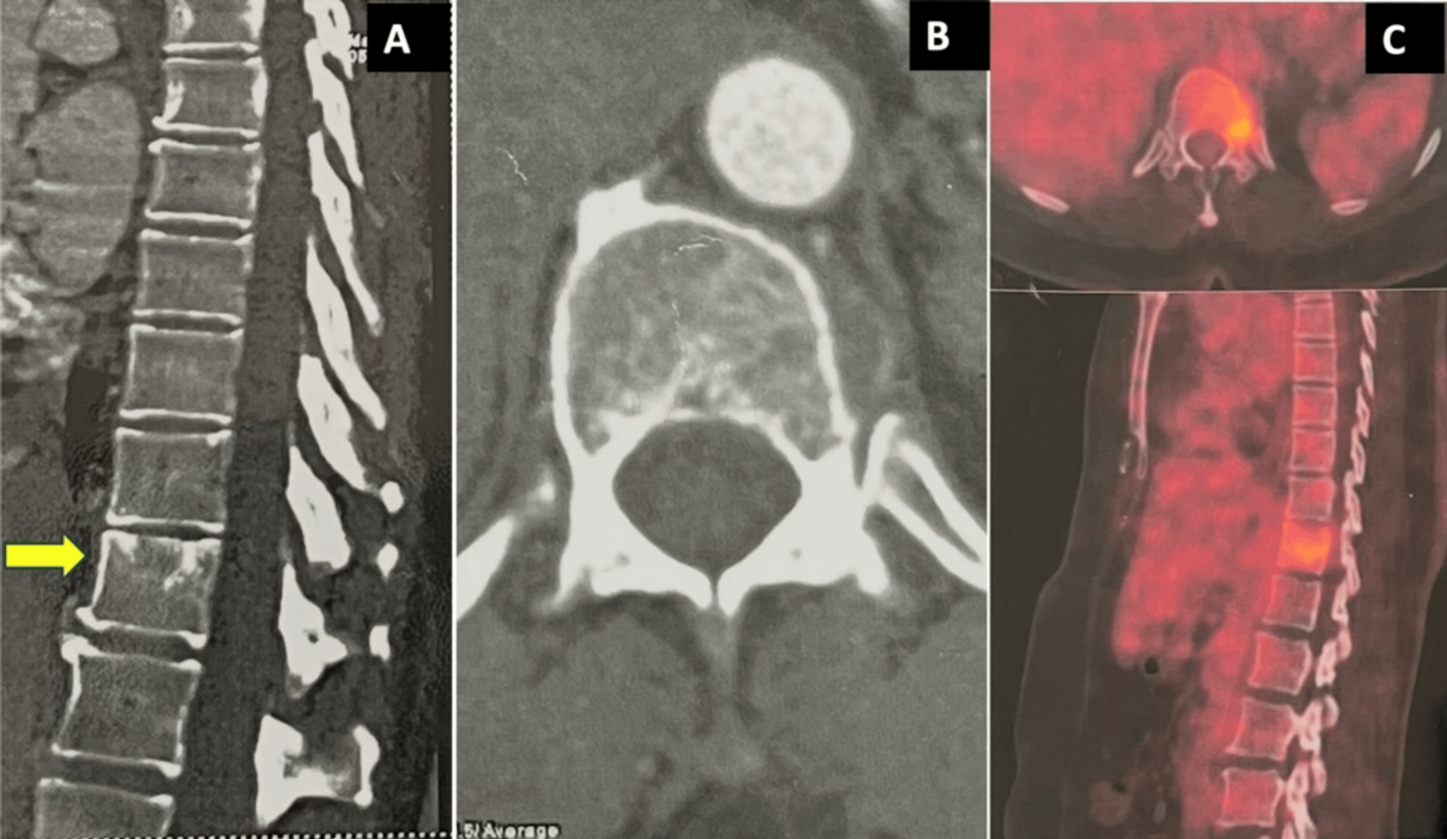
Preoperative CT and PET scans of the patient. The CT scan shows the mid-sagittal cut (A) with the T11-involved vertebra (yellow arrow) and the axial cut (B) of the involved vertebra without any significant findings. The PET-CT scan (C) shows increased uptake in the index vertebra. CT: computed tomography; PET: positron emission tomography
